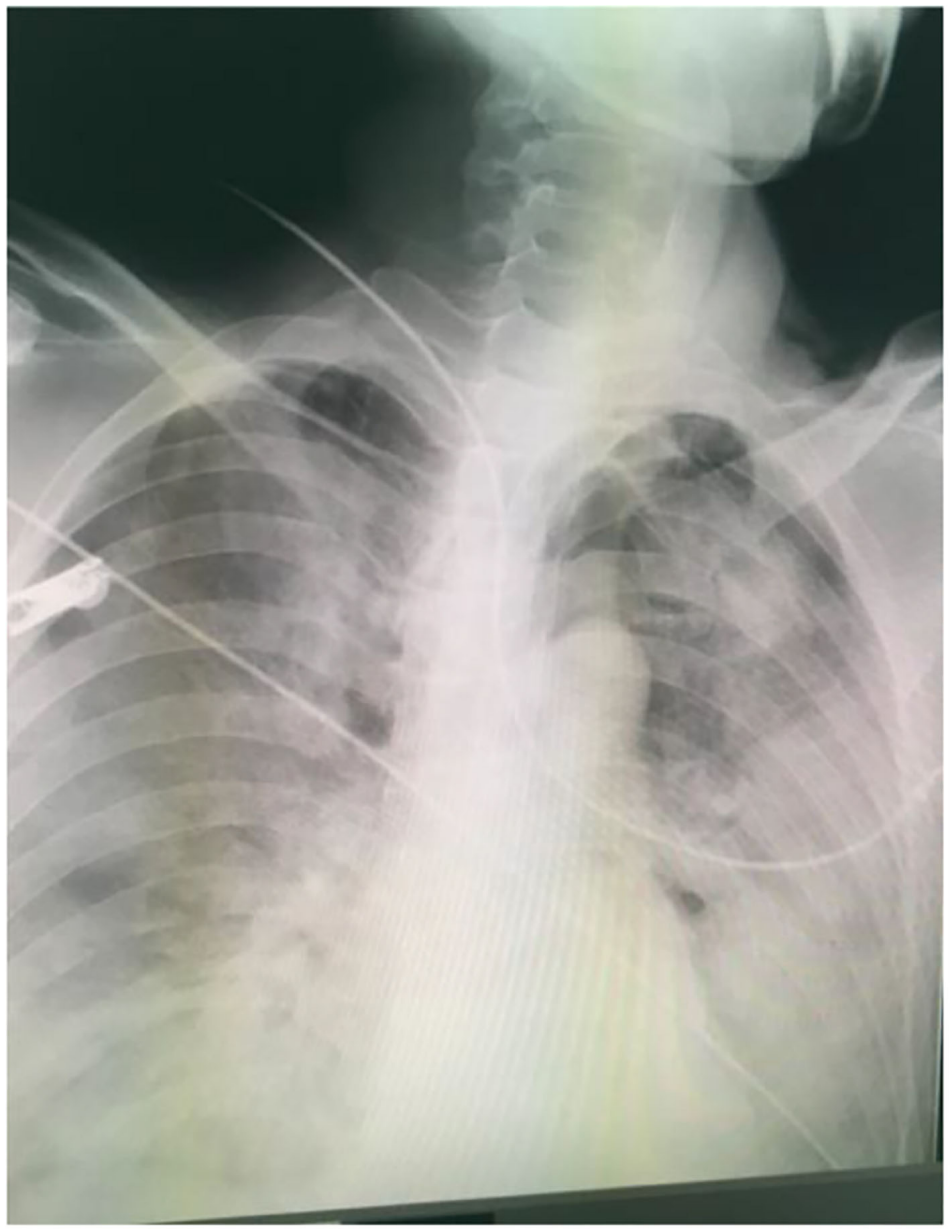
Chest x-ray revealed pulmonary edema with fluid-filled bilateral lungs.
